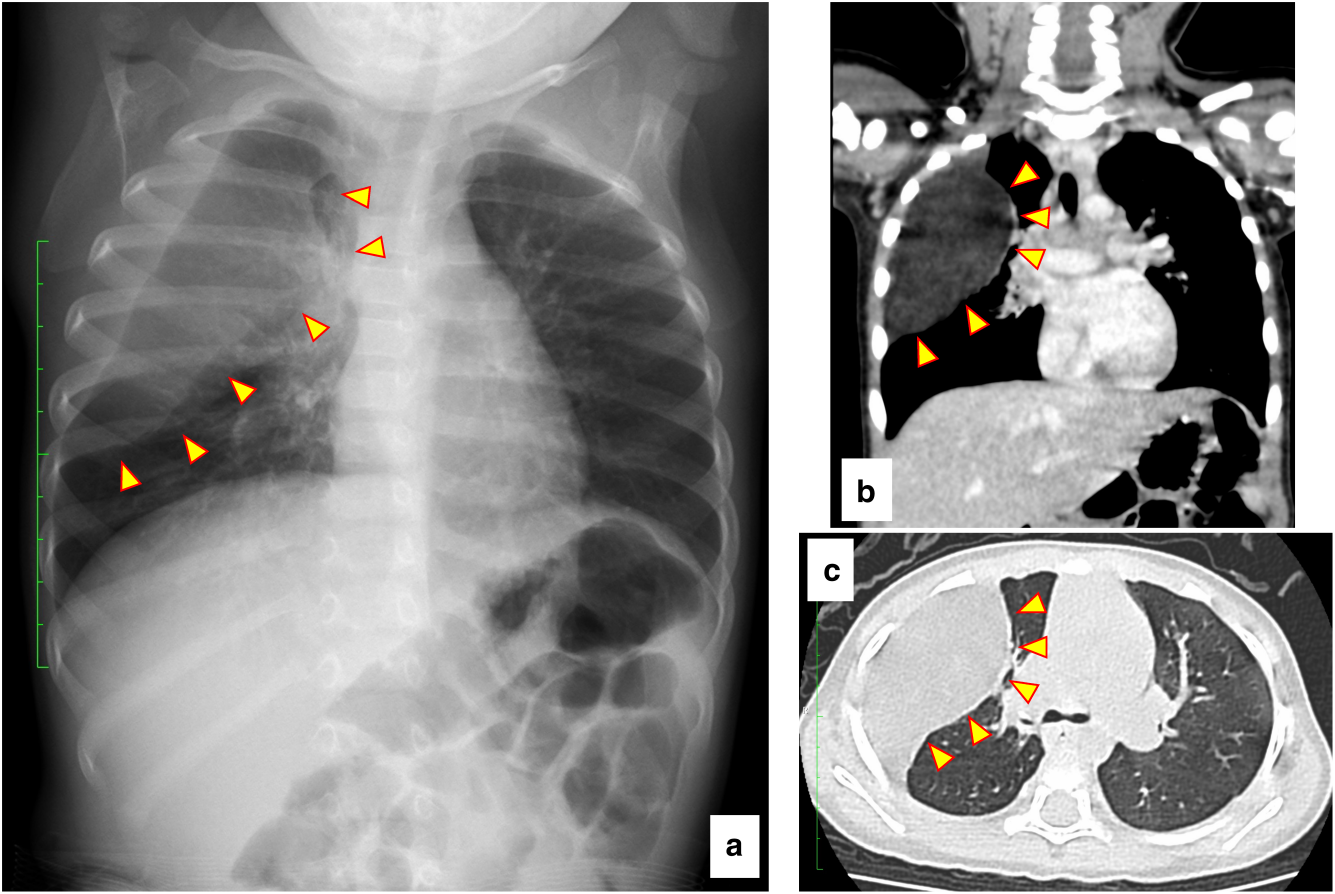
(a) Radiograph of the chest showing a convex lens‐shaped shadow extending from the right chest wall (yellow arrowheads). (b) Axial CT image: A tumorous lesion measuring 80 × 41 × 72 mm is visible along the chest wall in the right thoracic cavity (yellow arrowheads). (c) Coronal CT image: A convex lens‐shaped lesion is visible along the chest wall in the right thoracic cavity (yellow arrowheads). CT, computed tomography.
